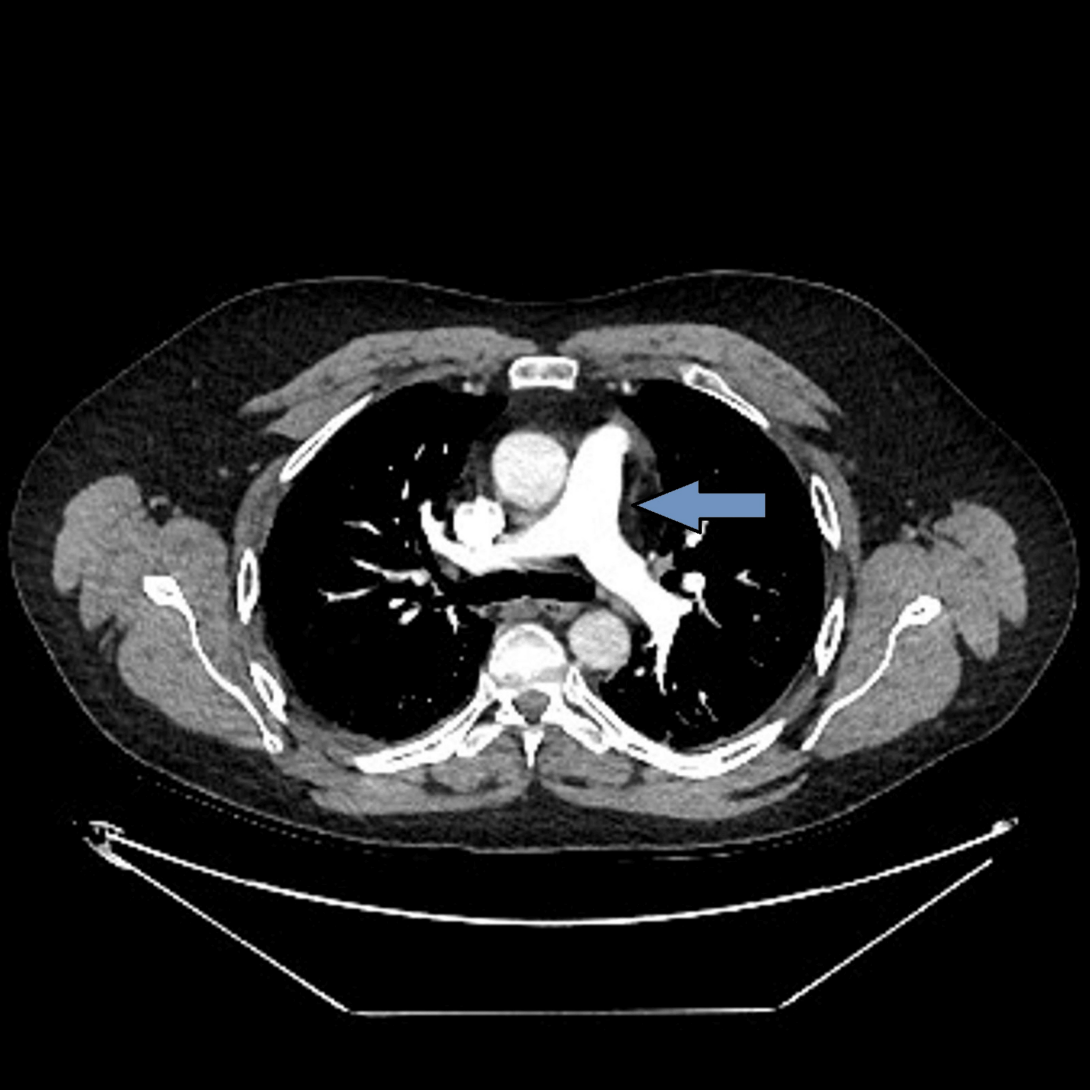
CT pulmonary angiogram axial image demonstrating well-opacified main and proximal pulmonary arteries with no intraluminal filling defects, ruling out central pulmonary embolism.
